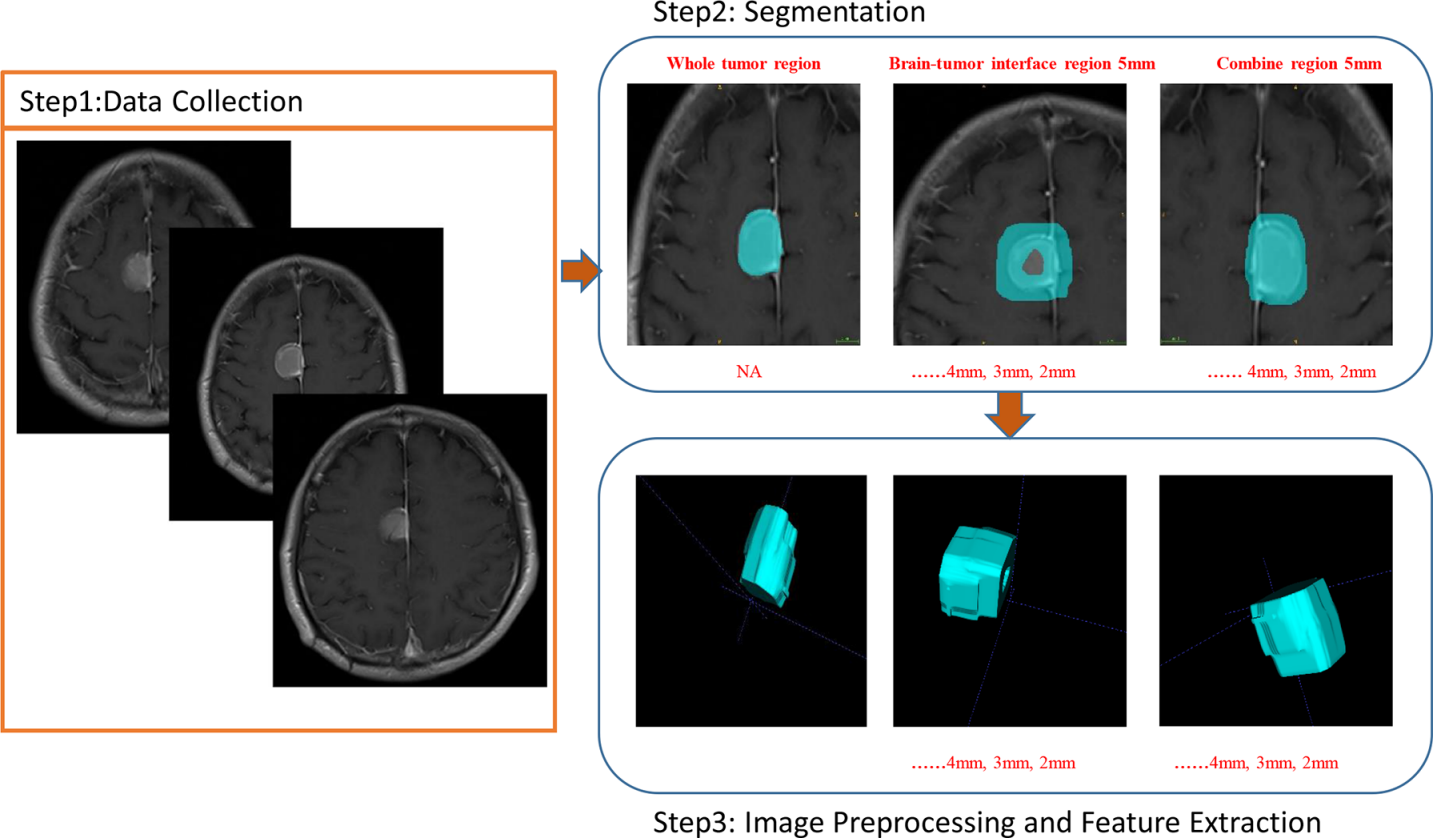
Images show the BTI, WT, and Com radiomics of invasive meningioma at CE-T1W MR image.
